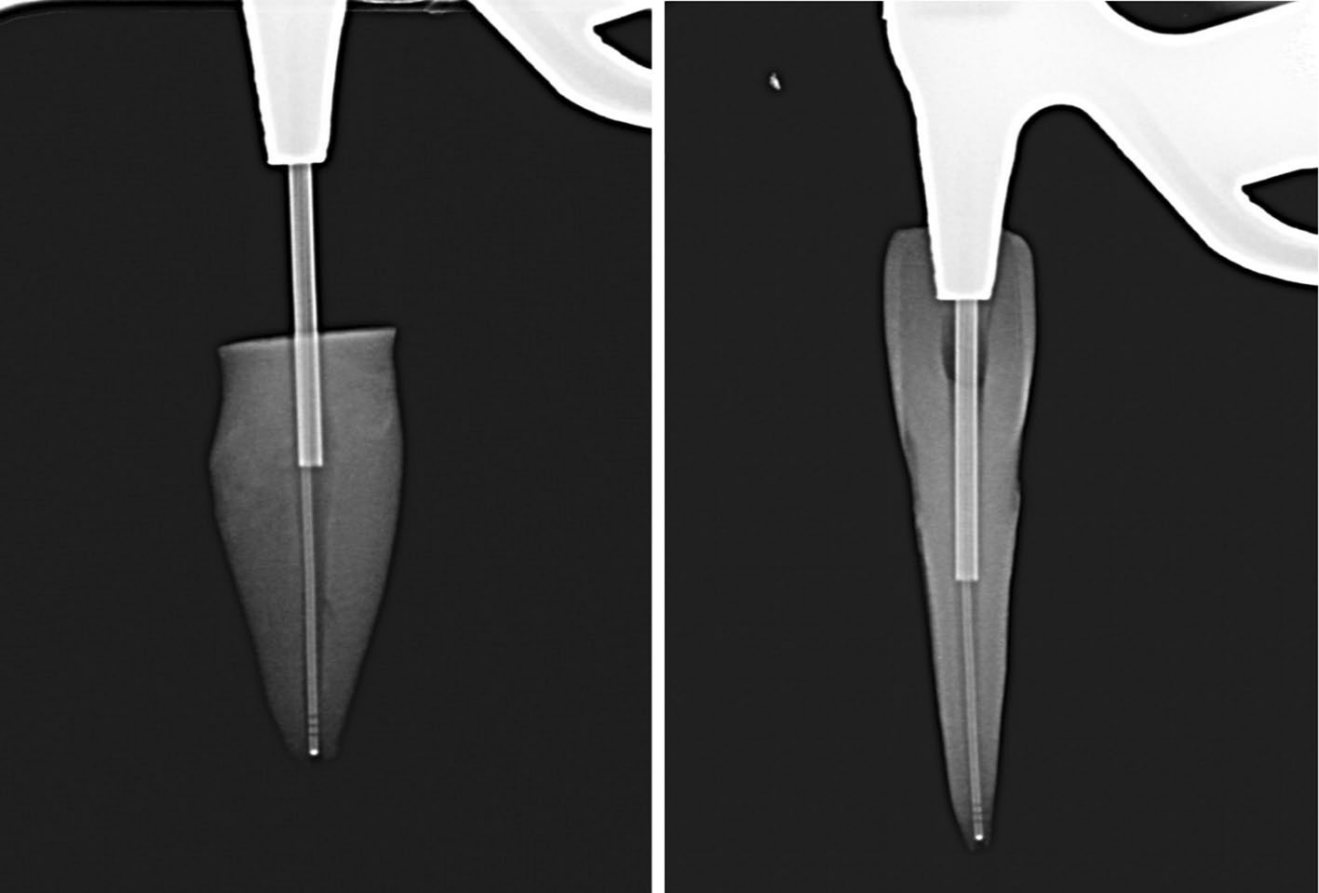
X-rays showing the device in the root canal and the micro-cannula reaching working length (showing both decoronated and non-decoronated roots).
